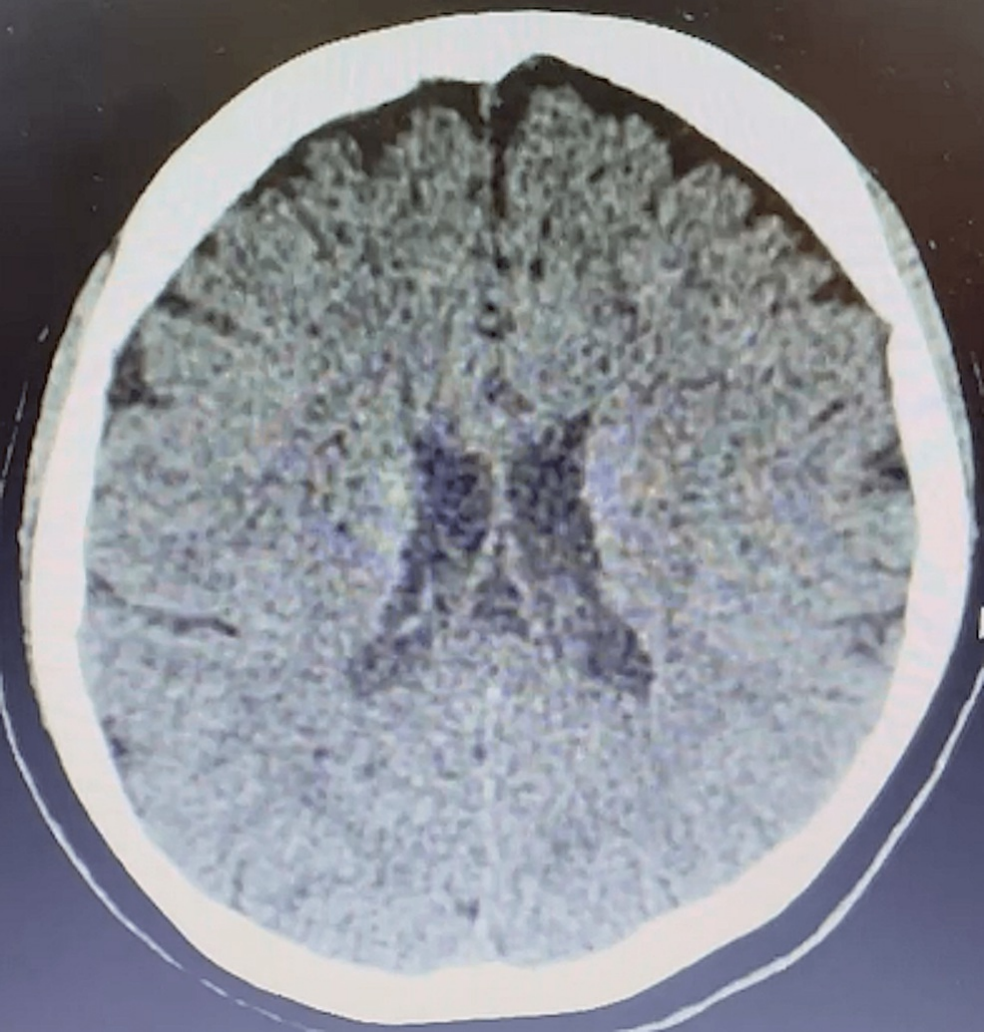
Contrasted computed tomography imaging of the brain revealed normal findings
